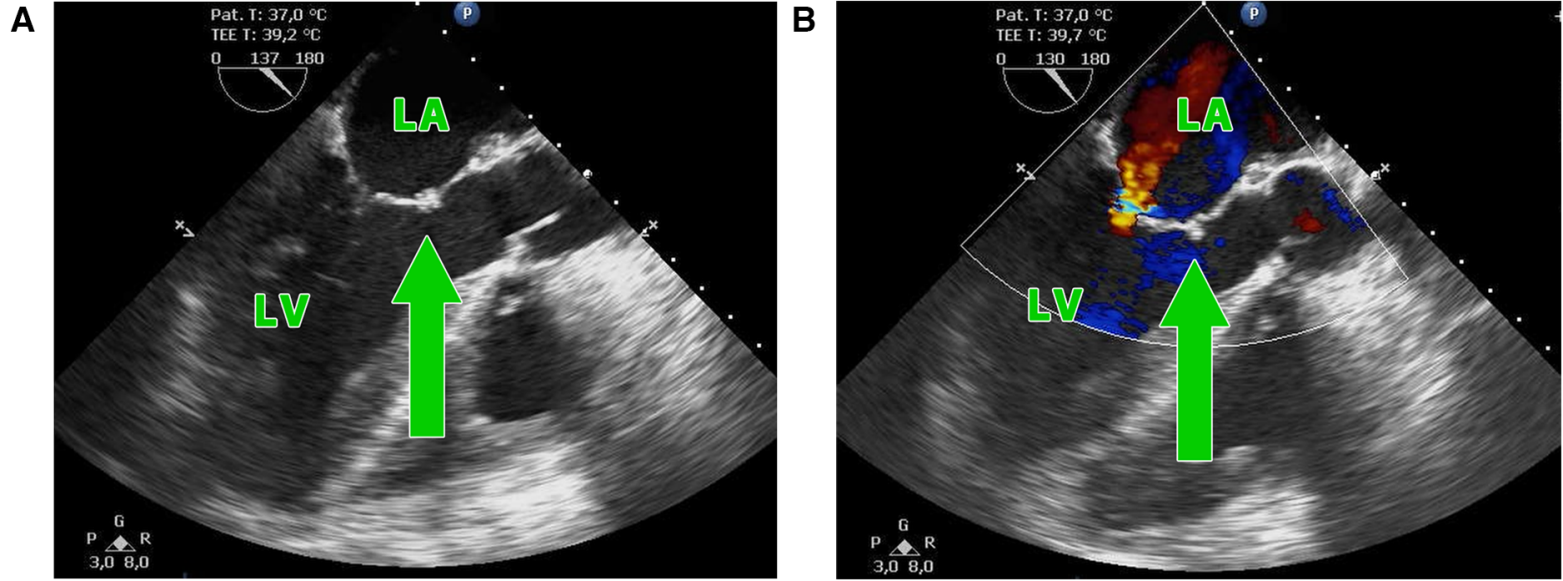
(A,B) Postoperative transesophageal echocardiography showing the suture of the anterior mitral leaflet of the homograft with the free edge of the patient's native anterior leaflet (green arrow) with residual mitral insufficiency. LA, left atrium, LV, left ventricle.
